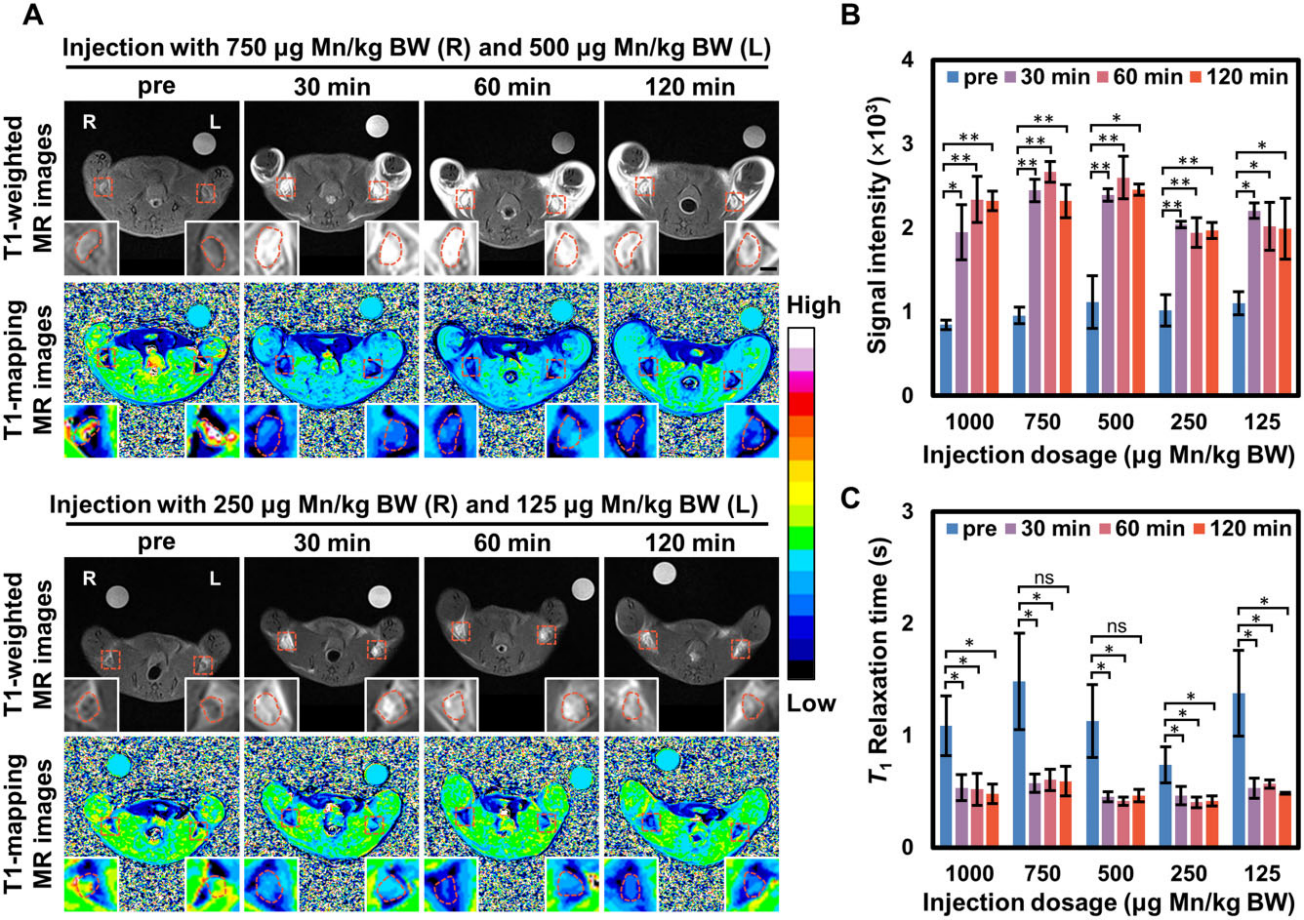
(A) *T*_1_-weighted MR images and *T*_1_-mapping pseudo-color images of popliteal lymph nodes before and after injection subcutaneously of MnC-20 at the dosage of 750, 500, 250 and 125 μg/kg BW, respectively (scalar bar = 1 mm); quantitative analysis of the signal intensity from *T*_1_-weighted MR images (B) and *T*_1_ relaxation time from *T*_1_-mapping images (C), and the data for 1000 μg Mn/kg BW were reproduced from Fig. 4. P* < 0.05; *P* < 0.01.
